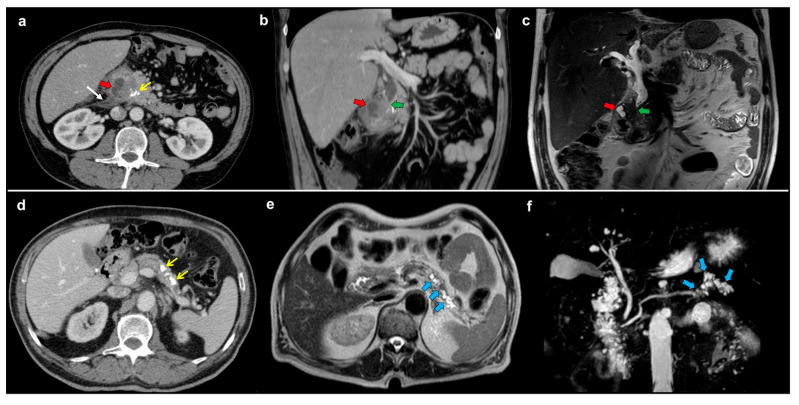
Two examples of chronic pancreatitis on CT and MRI. (a,b) Axial and coronal pancreatic-phase CT and (c) coronal T2WI show findings consistent with chronic groove pancreatitis, including calcifications (yellow arrow), cystic dystrophy of the duodenal wall (red arrow) and marked narrowing of the intrapancreatic CBD (green arrow). Stranding of the adjacent fat with a small amount of free fluid in the right anterior pararenal space is also present (white arrow), suggesting an acute exacerbation. (d) Axial portal-phase CT, (e) axial T2WI and (f) coronal 3D MRCP with MIP reconstruction show diffuse chronic pancreatitis, characterised by parenchymal atrophy, ductal lithiasis and calcifications (yellow arrows), as well as irregular dilatation of the MPD with intermittent strictures (blue arrows).
